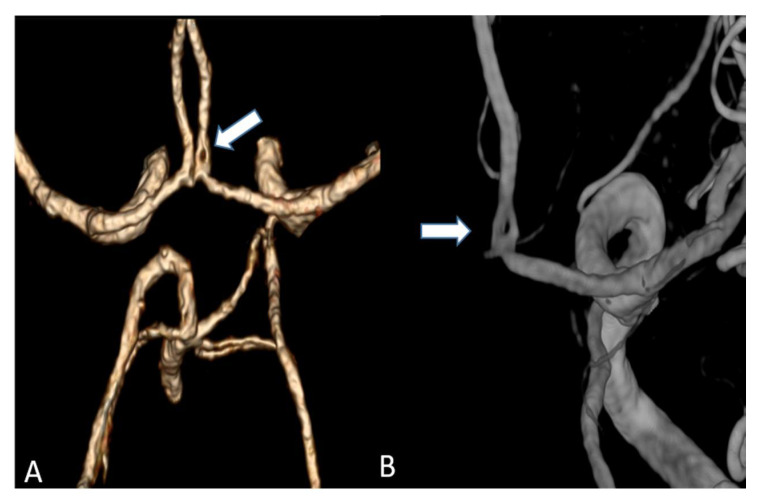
(A) Volume rendering of an anterior cerebral artery fenestration acquired by an UHR-CT (B) Three-dimensional (3D) digital subtraction angiography (DSA) to confirm arterial fenestration in (A), which is often not detected by normal resolution CTAs.
